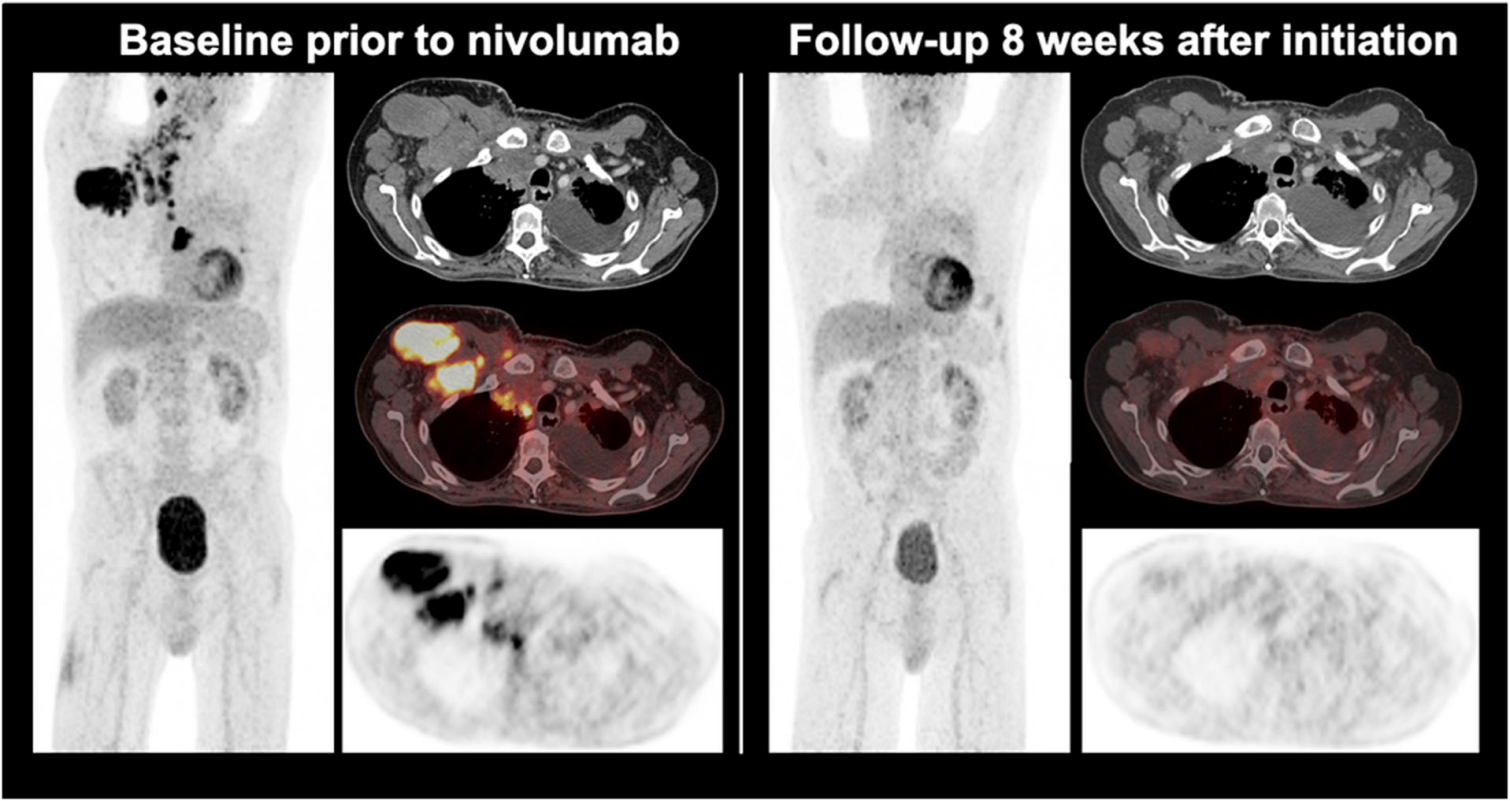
A Hodgkin lymphoma patient with metabolically active tumour manifestations prior to the initiation of immunotherapy with nivolumab. A complete metabolic response already 8 weeks after immunotherapy initiation despite remaining morphological masses on CT was observed
